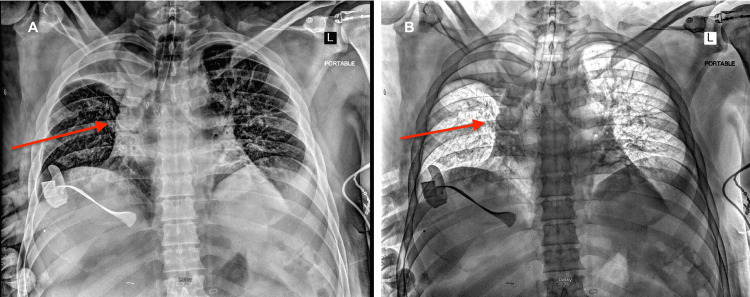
Chest radiography without inversion (A) and with inversion (B). Portable chest radiographs demonstrating an abnormal opacity involving the right upper chest wall region (red arrows), corresponding to the location of the invasive chest wall tumor seen on CT imaging. Figure 1B is shown in inverted contrast to better delineate the invasive chest wall tumor.
